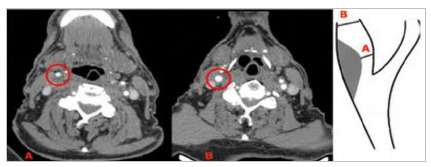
CT angiography of right internal carotid stenosis greater than 70%. A: transverse section at the point of greatest stenosis. B: transverse section at the point of normal artery distal to the stenosis (NASCET stenosis: BA/B*100
